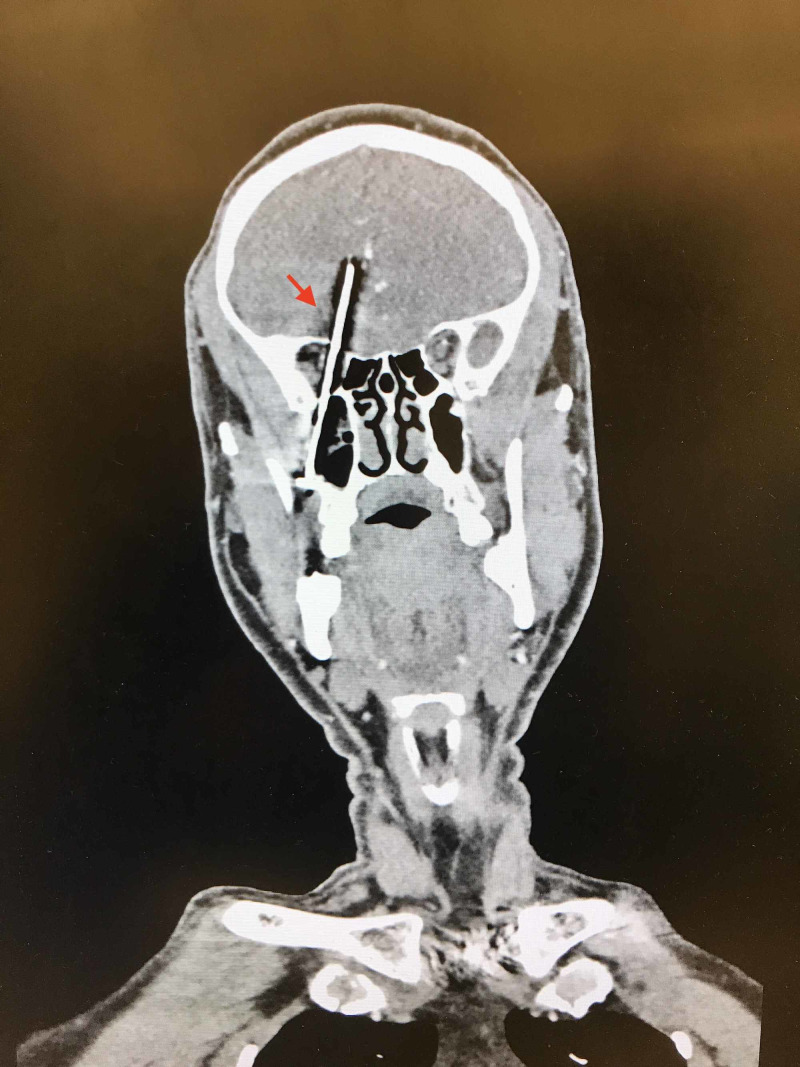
Coronal CT demonstrating penetration of the anterior skull base, demonstrating proximity to the anterior cerebral artery (ACA)
